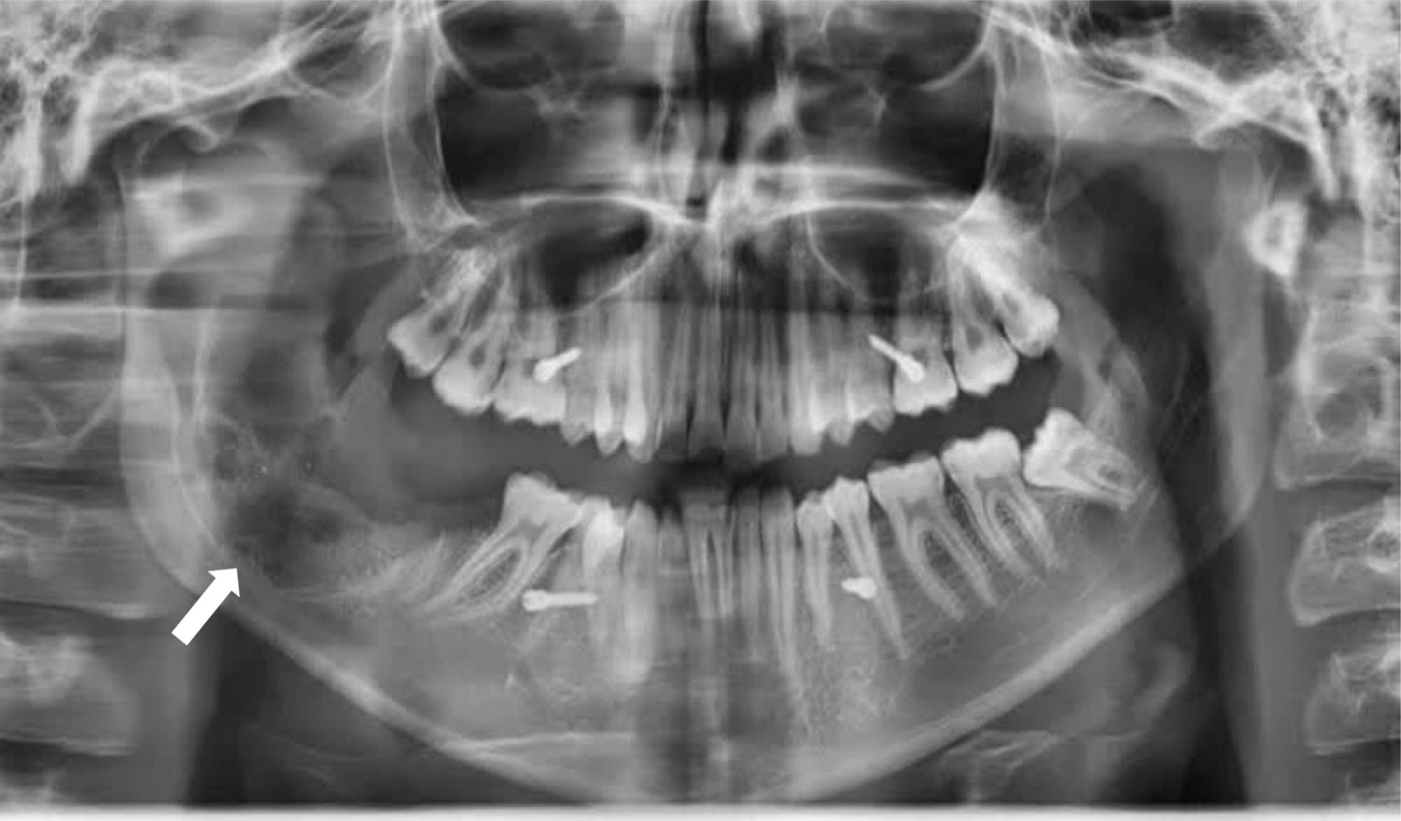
Follow-up panoramic radiograph obtained one month after surgery, showing a significant reduction in the multilocular radiolucency previously associated with the right mandibular third molar (#48), with no further progression of resorption in the adjacent right second molar (#47), indicating a favorable healing response (white arrow).
